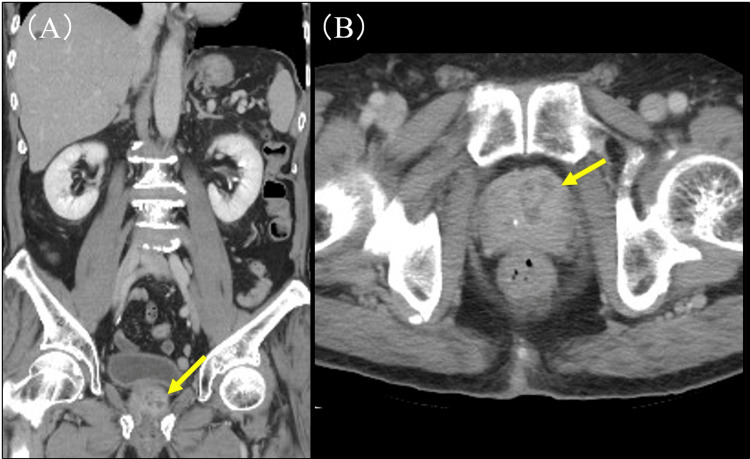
Results of contrast-enhanced CT. (A, B) Abdominal CT showing a low-density area in the prostate gland. CT: computed tomography
